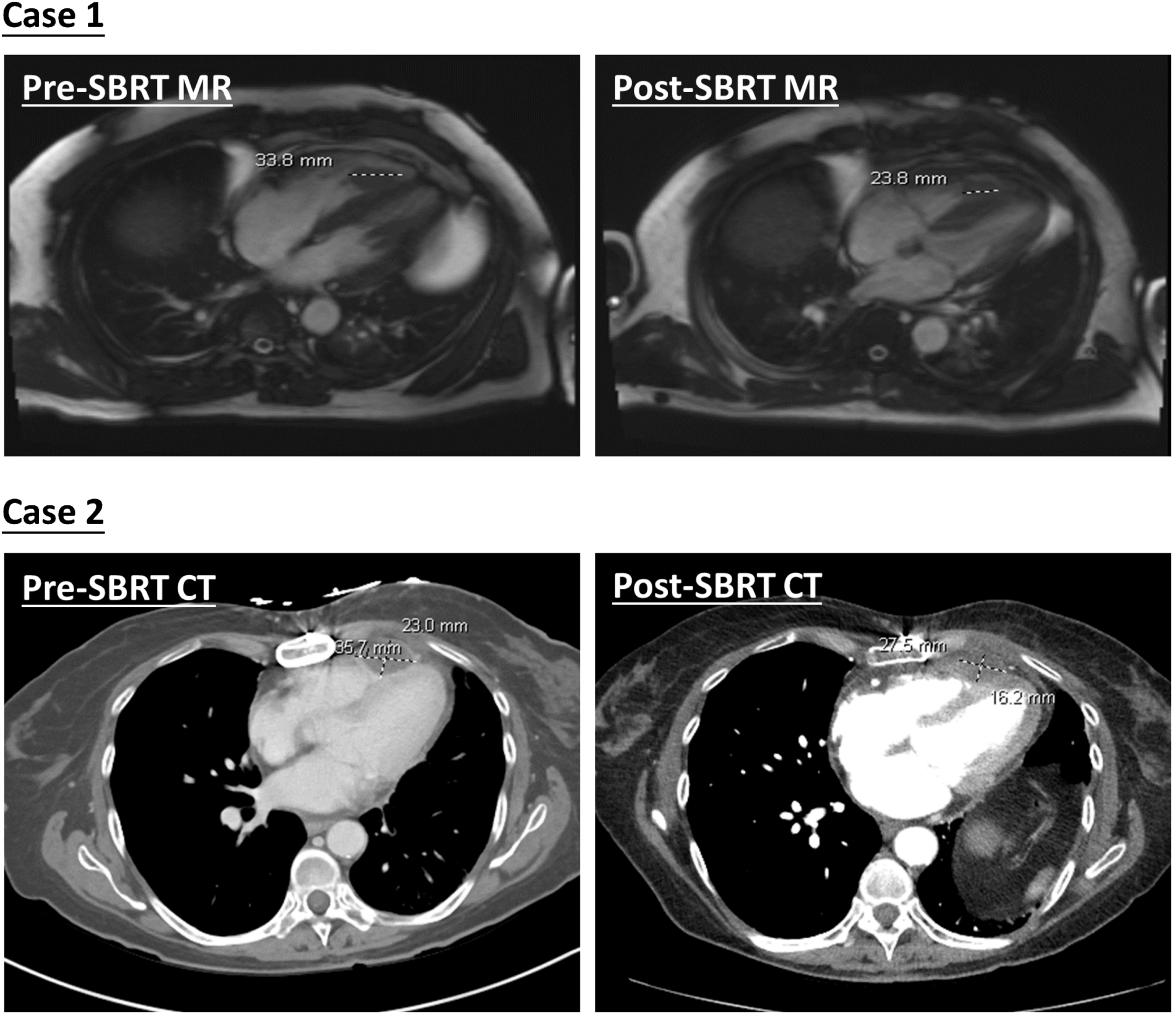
Demonstration of pre- and post-SBRT cardiac lesions. Diagnostic MR and CT images were both acquired with contrast agents.
